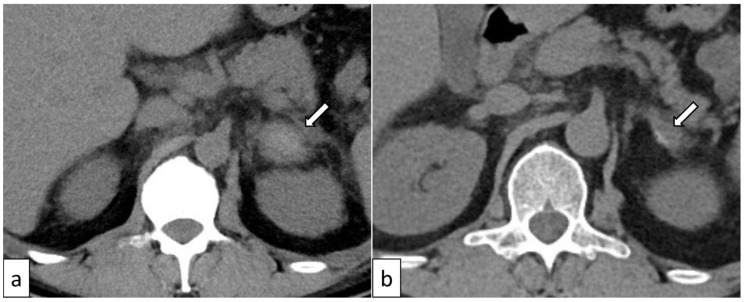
A 50-year-old man with TAFRO syndrome. A non-enhanced CT shows an enlarged and high-attenuated left adrenal gland, indicating adrenal hemorrhage ((a), arrow). Ten months later, the atrophy and calcification of the affected adrenal gland were observed ((b), arrow). CT images of the different parts of the body were evaluated in a previous study [15].
